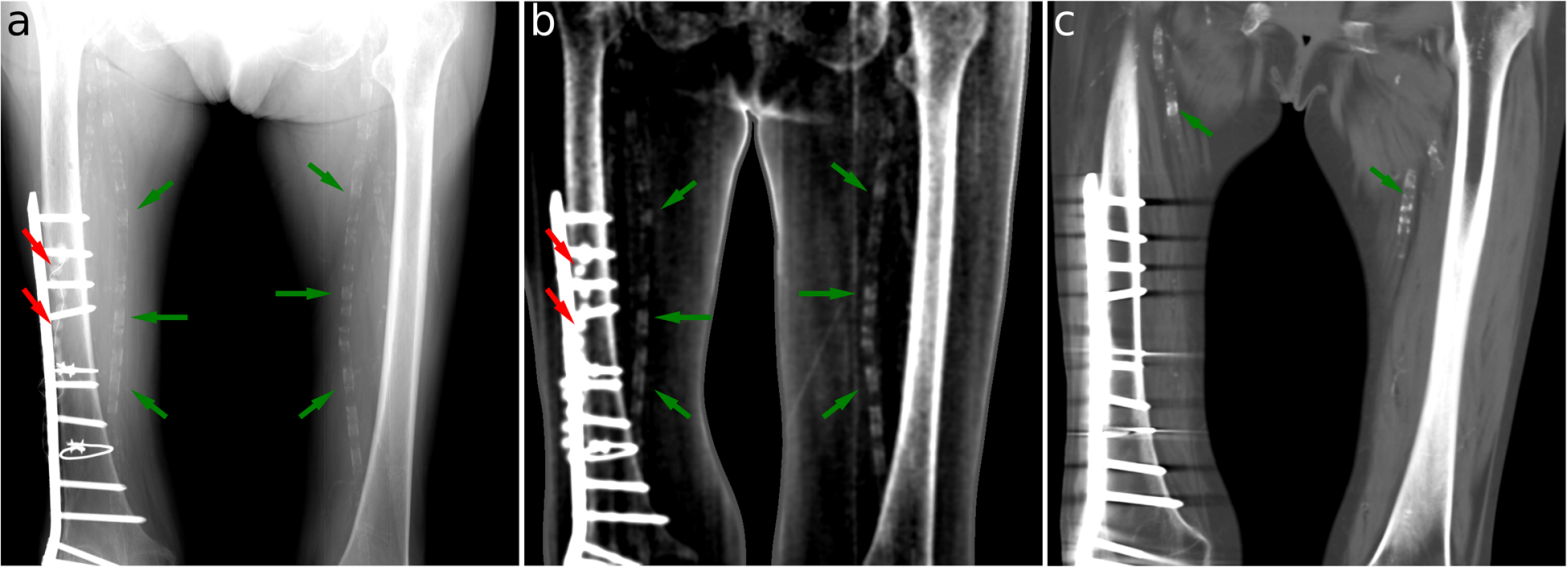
X-ray attenuation (window level 2.45, window width 1.9) (a), dark-field (window level 0.4, window width 2.0) (b) radiographs, and a computed tomography reconstruction (c) of the upper legs. The metal screws and plate on the right femur, a fixation of an old fracture, are clearly visible in all three modalities. Near the screws, an antibiotic bead chain is also visible in the attenuation and dark-field images (red arrows). Furthermore, calcifications are visible within the superficial femoral arteries in both thighs at all three modalities (green arrows)
